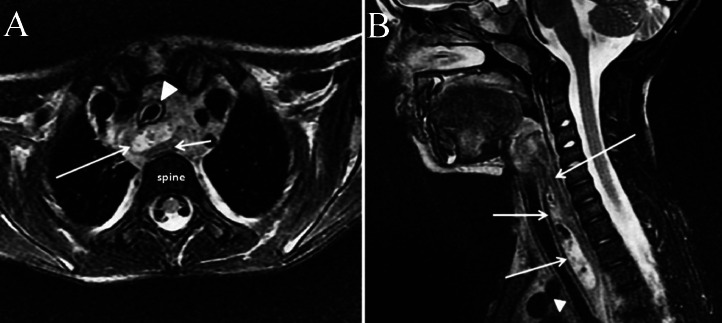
MRI of the neck. (A) In the axial view images shows an abscess (long arrow) which compresses esophagus posteriorly (short arrow) and trachea anteriorly (head arrow). (B) The sagittal view images show better the craniocaudal extent of abscess (short arrows) and the relationships it has with to the esophagus (long arrow) and trachea (arrow head).
